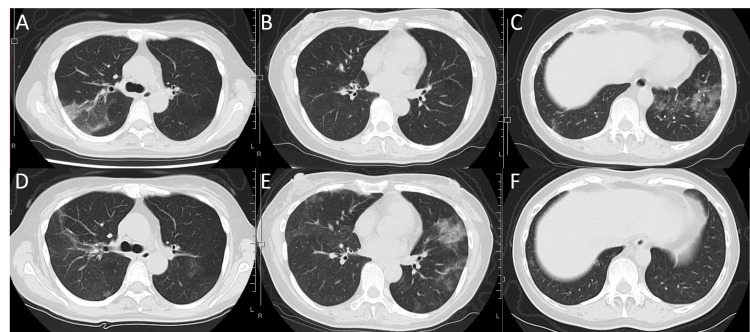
Chest CT in case 1 Chest CT one month before the first consultation showing ground-glass opacities (GGOs) in the right superior lobe on pleural side (A) and left inferior lobe(C) of the lung. The lingular lobe of the left lung is clear (B). Repeat chest CT at the first consultation showing improvement of opacities that were previously observed in the right superior lobe (D) and left inferior lobe (F) of the lung. New opacities were detected in the lingular and inferior lobes of the left lung (E).
